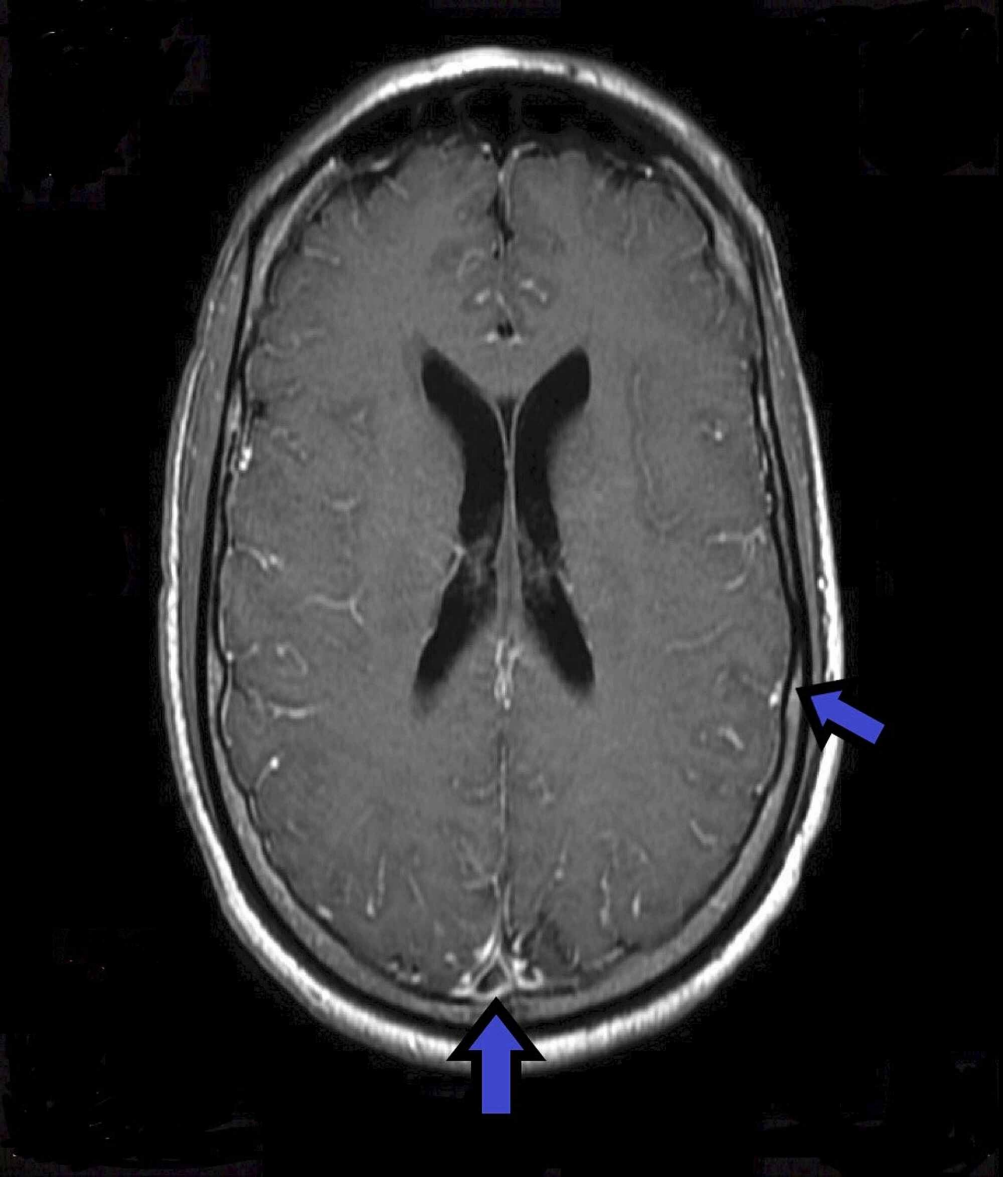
Magnetic resonance images of the brain with and without contrast showing meningeal enhancement but no basal ganglia lesion Blue arrows indicate meningeal enhancement.
